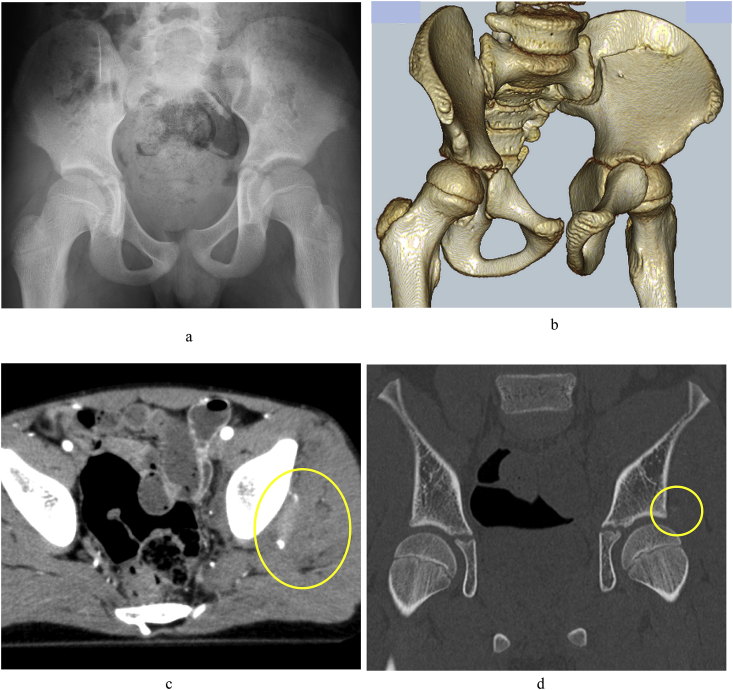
a) X-ray at the time of injury. Pubic symphysis diastasis can be seen. b) Three-dimensional CT showing left sacroiliac joint widening. Left iliac wing fracture can be seen. c) CT horizontal section. Small bone fragments and hematoma can be seen in the lateral side of the left acetabulum. d) CT coronal section showing small bone fragments on the lateral side of the left acetabulum.
